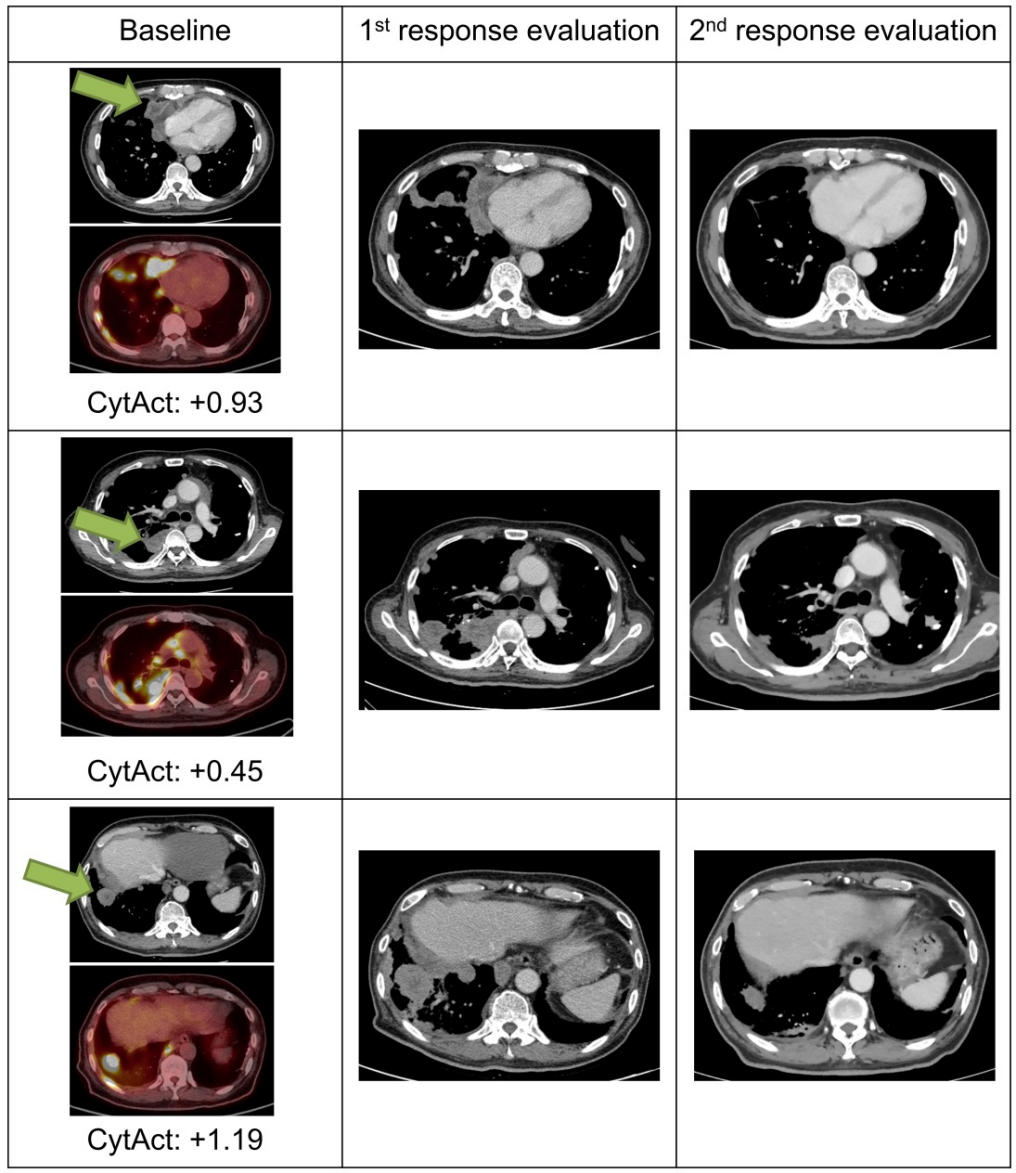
Images and predicted CytAct of a pseudo-progression case. A 69-year-old female patient diagnosed with LUAD underwent 2 cycles of pemetrexed and cisplatin, after which the cancer progressed as shown in the images of the baseline column. The patient then received pembrolizumab, and 1^st^ response evaluation was done after 1 month. Although the 1^st^ evaluation CT scan showed progression, the patient continued pembrolizumab because the general condition of the patient was clinically improving. After 2 more months, a 2^nd^ response evaluation CT scan was done and showed regression of tumor masses. The target lesion is marked with green arrows in the figure. Predicted CytAct values as shown below the PET images were higher than 0.107, the value that discriminates responders from nonresponders described later in the manuscript.
